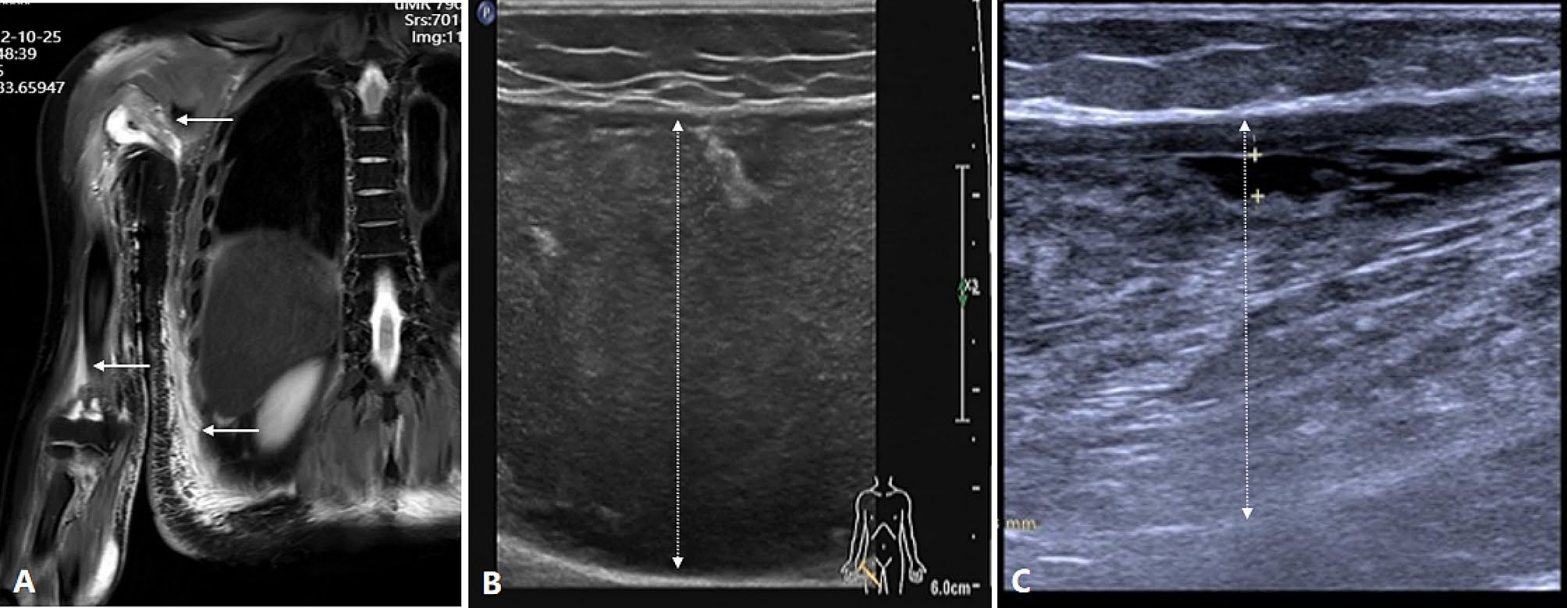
MRI of subcutaneous abscesses in the upper right limb and waist (A). The sites of involved muscle with high signals were defined by white arrows. Ultrasound revealed signs of inflammation and local fluid in left thigh (B), and the abscess faded obviously after treatment for 2 months (C)
